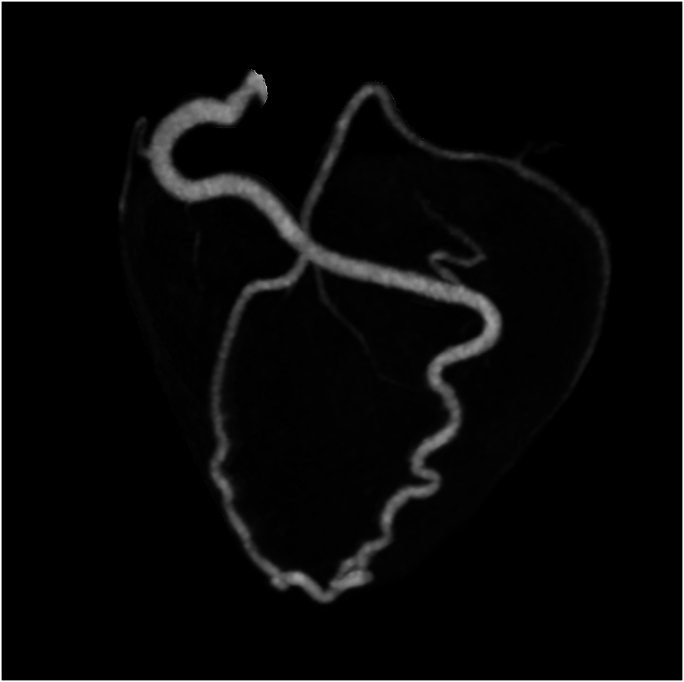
A pre-operative maximum intensity projection image of a coronary computed tomography. The right coronary artery and LAD are connected directly in the distal segment.
